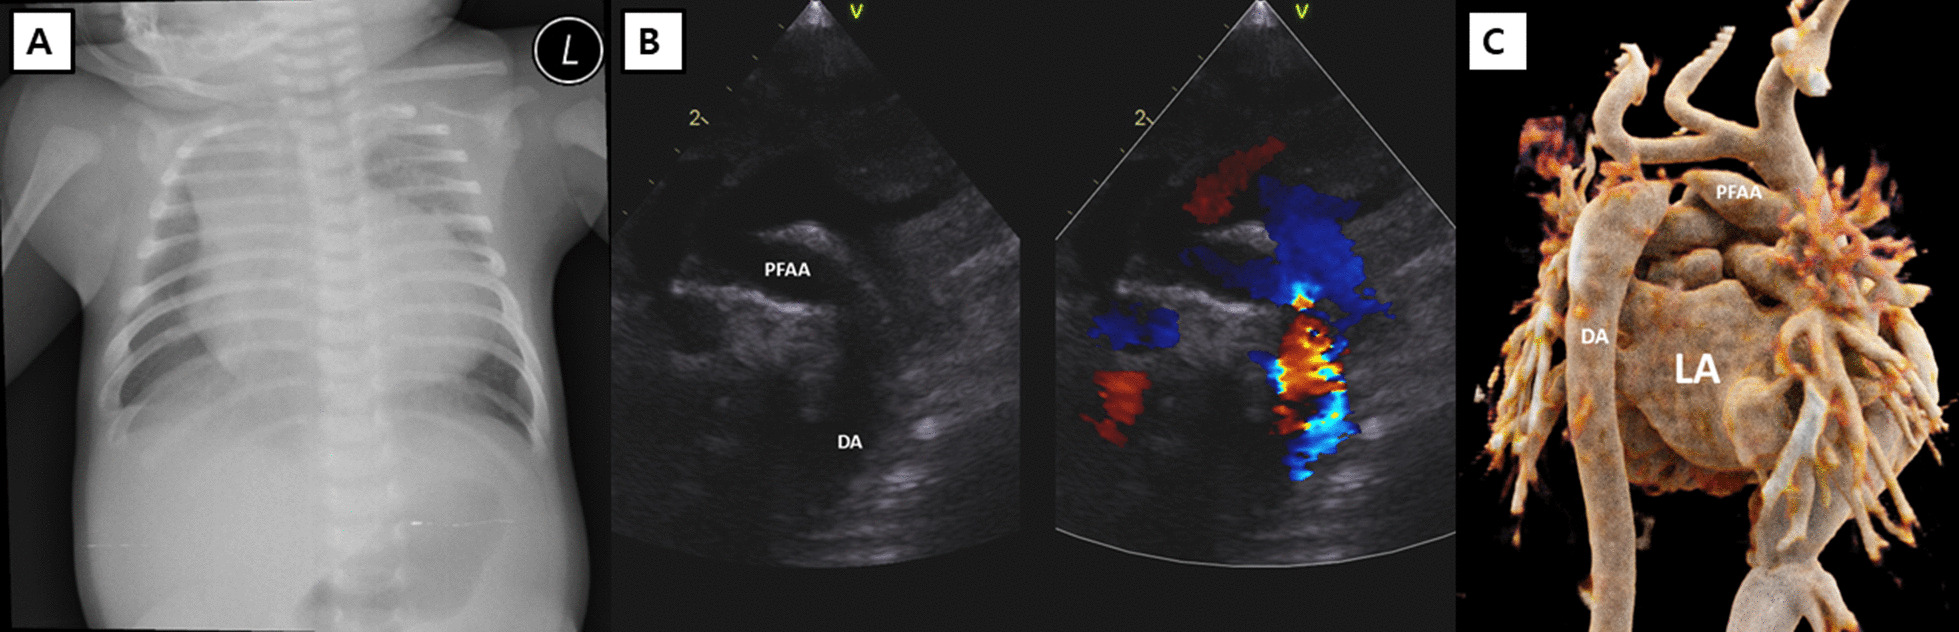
Severe cardiomegaly was identified on the initial chest x-ray (a), and preoperative echocardiography (b) and three-dimensional computed tomography angiography (posterior view) showed the persistent fifth aortic arch with severe aortic coarctation (c)
